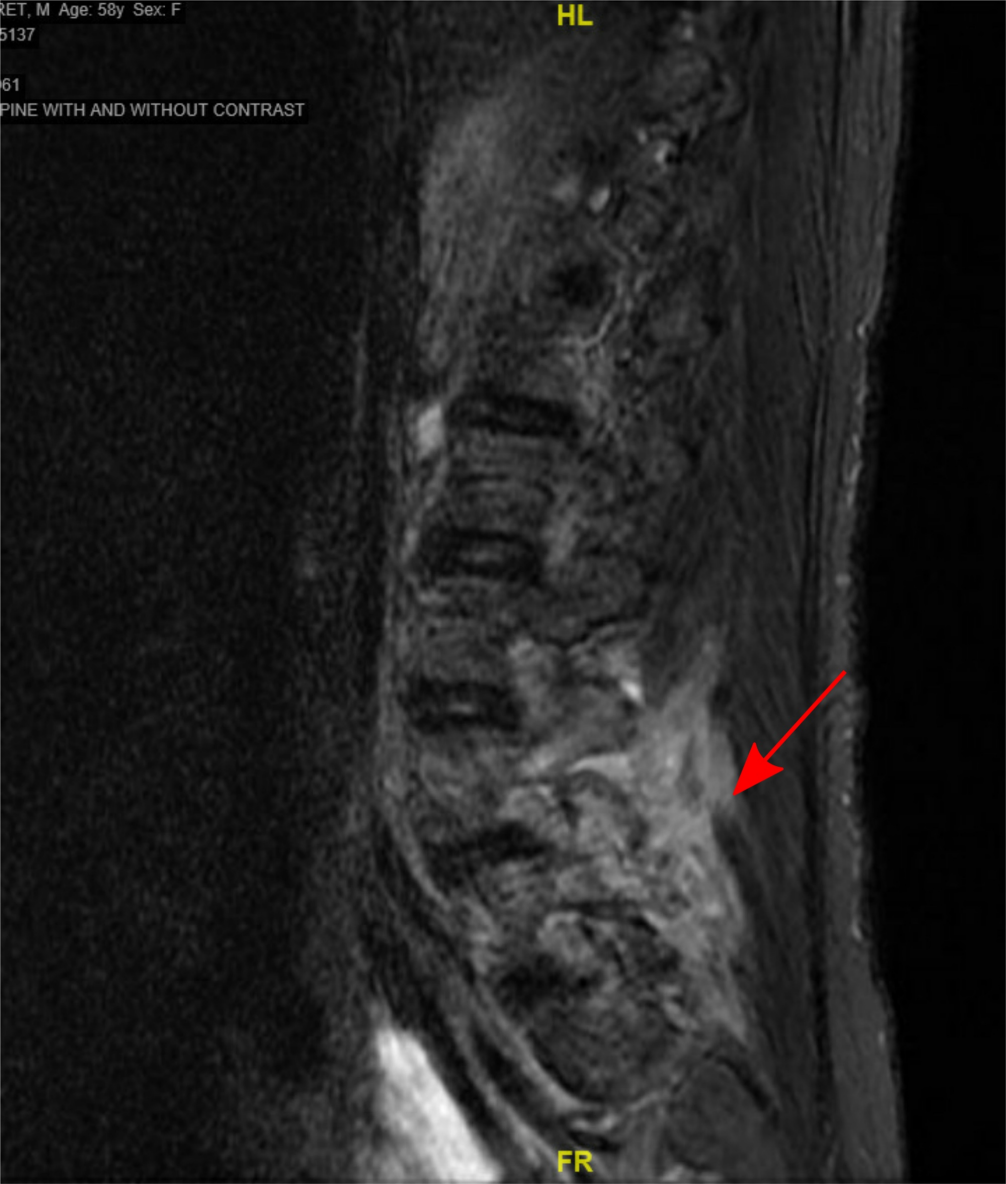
MRI of the lumbar spine showing epidural abscess (red arrow)
